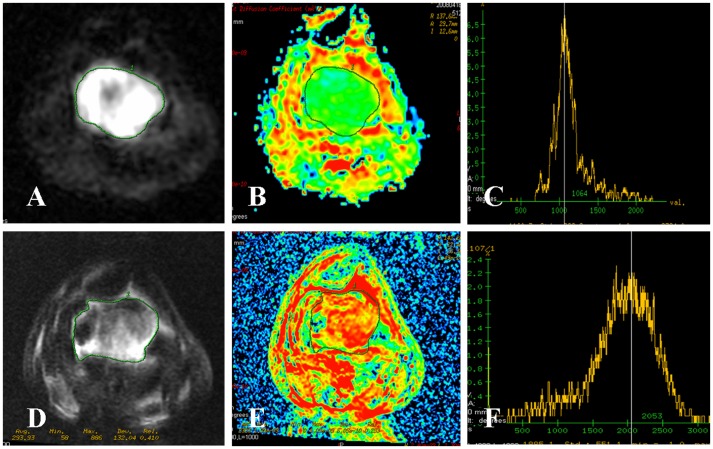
Osteosarcoma of the distal femur in a 24-year-old man with good response. (A∼C) DWI map, ADC map and ADC histogram before neoadjuvant chemotherapy. The signal intensity of tumor on DWI map was high. The ADC value of the whole tumor was 1.12×10^−3^ mm^2^/s with a green area. The ADC histogram was high and sharp. (D∼F) DWI map, ADC map and ADC histogram after neoadjuvant chemotherapy. The signal intensity of tumor on DWI map was decreased. The ADC value of the whole tumor was increased to 1.99×10^−3^ mm^2^/s with a subtotal red area. The ADC histogram was short, wide and moving to the right of the coordinate. Tumor necrosis rate of 92% was confirmed by postoperative pathological evaluation. The effectiveness of neoadjuvant chemotherapy was good.
